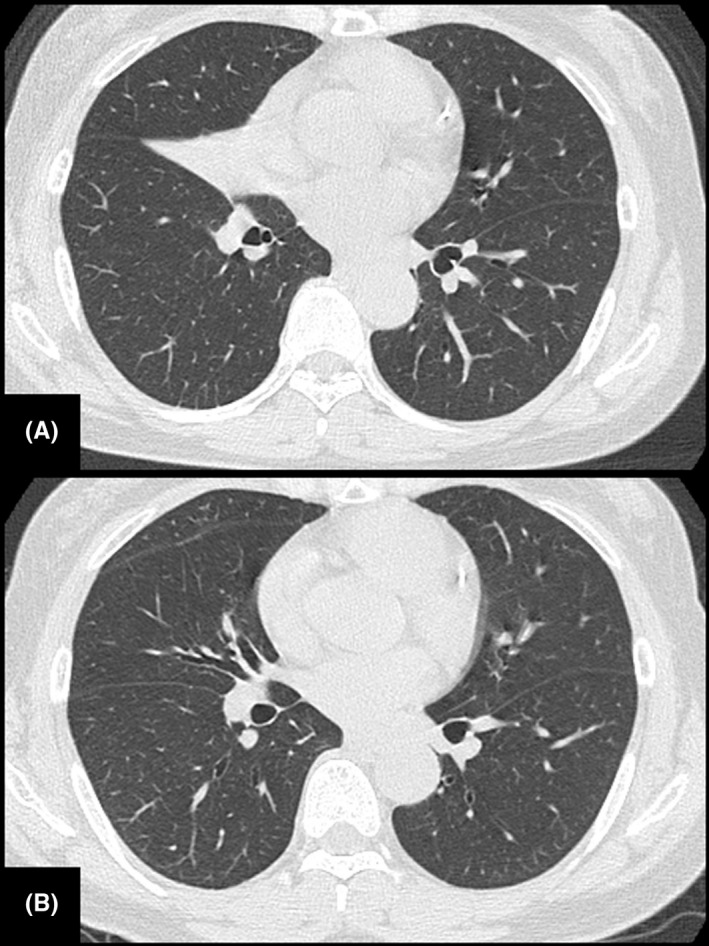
(A) Chest CT showed atelectasis of the middle lobe of the right lung. (B) The atelectasis resolved after the removal of a foreign body in the right middle lobe bronchus.
